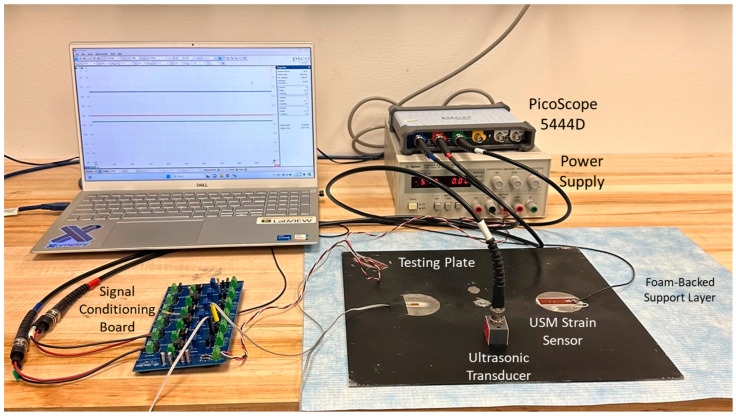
Acoustic emission experimental testing setup. Note that the ultrasonic transducer could be fixed to various locations depending on the desired setup.
